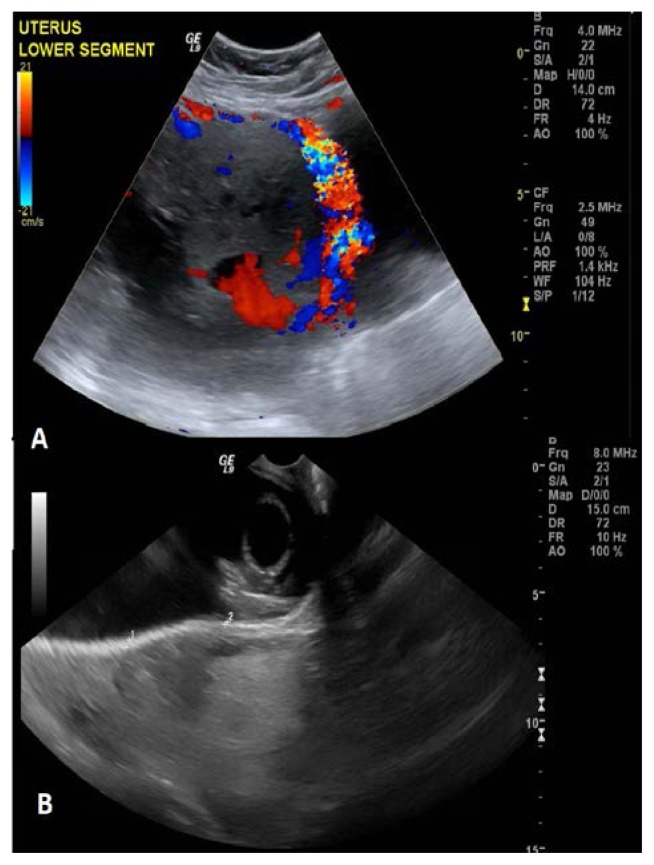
Clinical images: A. Doppler Ultrasound and B. Ultrasound of the Uterine Lower Segment
